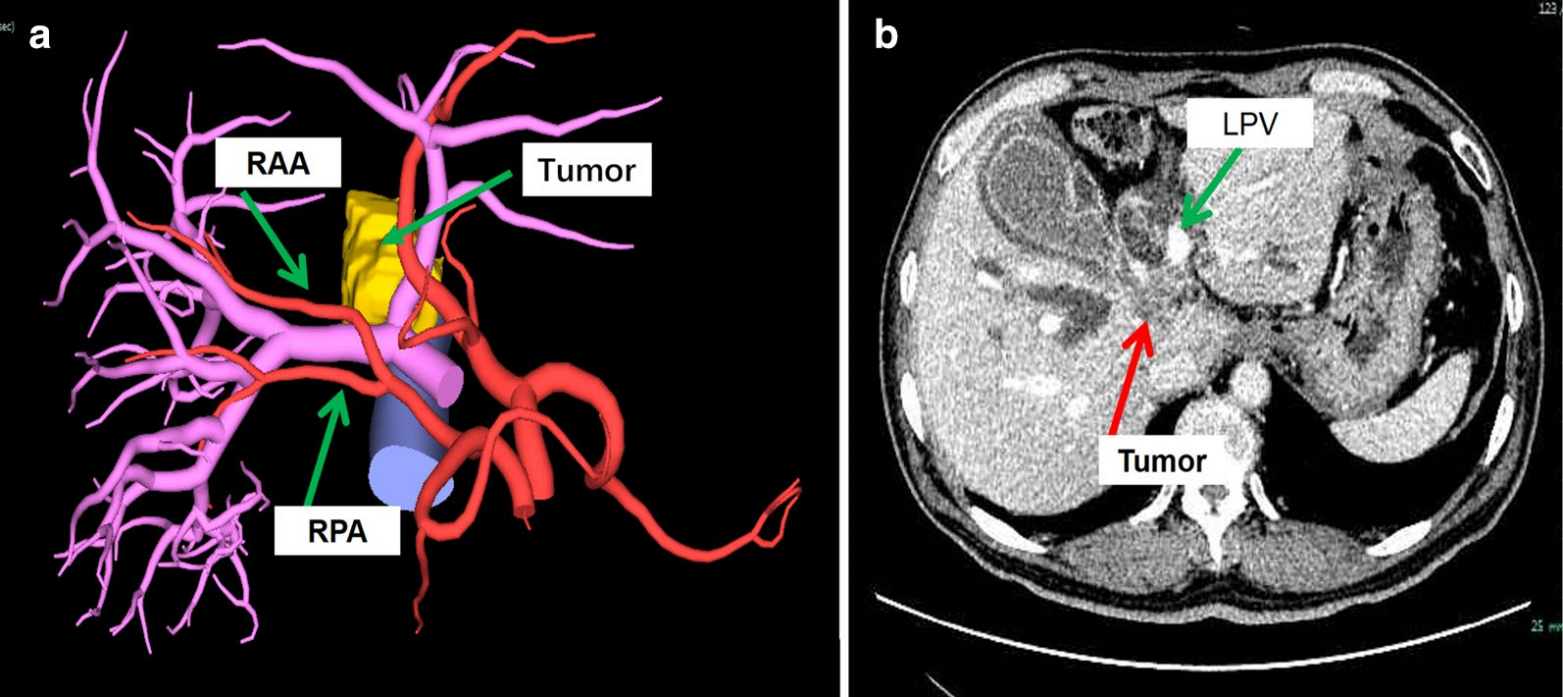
Location of tumor and vessels: a the three-dimensional reconstruction of the tumor and artery, the right branch was closely related to the tumor, and the right hepatic artery was from the superior mesenteric artery and was not affected. b The CT scan which shows the tumor was located at the bifurcation of the portal vein, the left branch of the portal vein was compressed. *RAA* right anterior hepatic artery, *RPA* right posterior hepatic artery, *LPV* left portal vein
